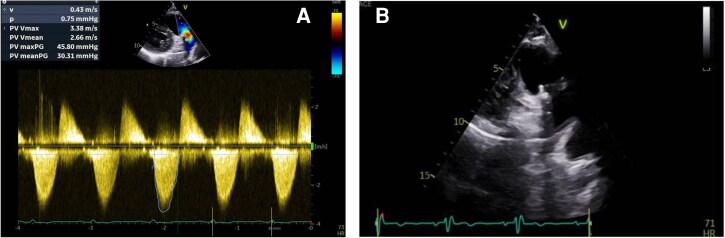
(*A*) parasternal short axis of the CW Doppler of pulmonary bioprosthesis. (*B*) Parasternal long axis right ventricular (RV) inflow showing the pulmonary bioprosthesis.
